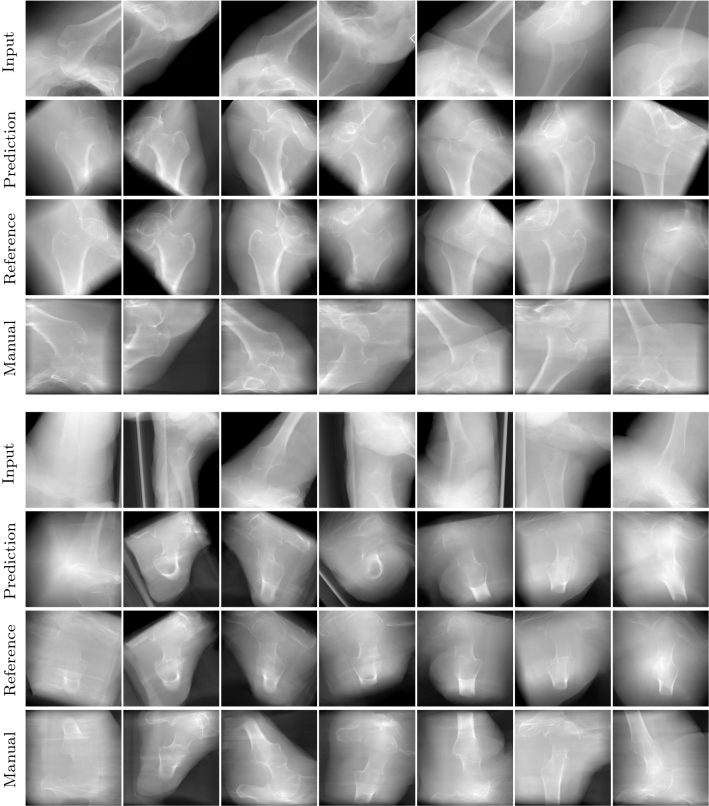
Qualitative evaluation of the pose regressor on real X-rays (first block: *AP* standard, second block: *Lateral* standard). For comparison, the last two rows show the reference standard defined by the expert in the C-arm volume and the manual standard acquired with the C-arm (image rotation implicitly defined by detector orientation)
